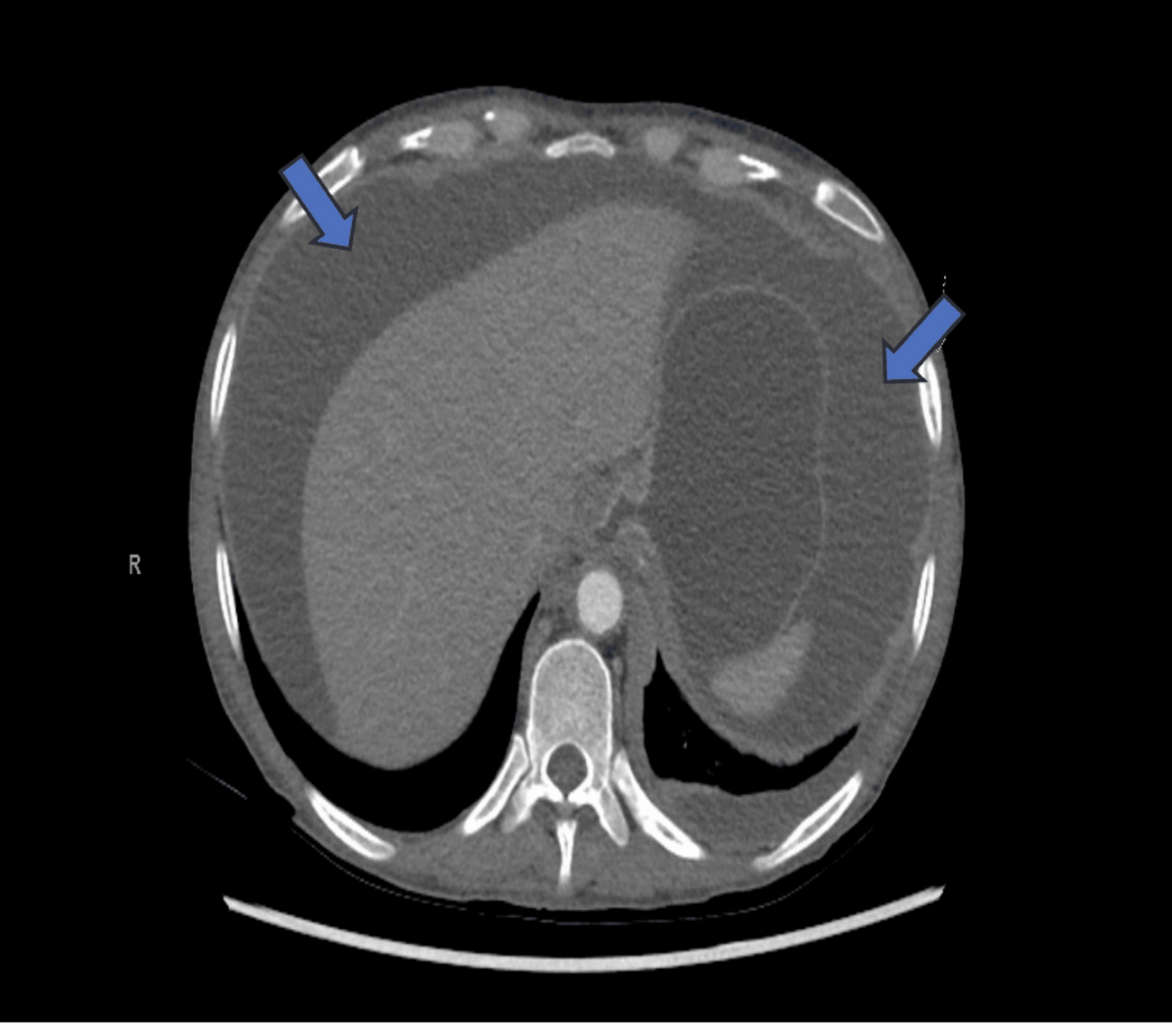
CT Abdomen showing large-volume abdominal ascites with normal appearing liver.
